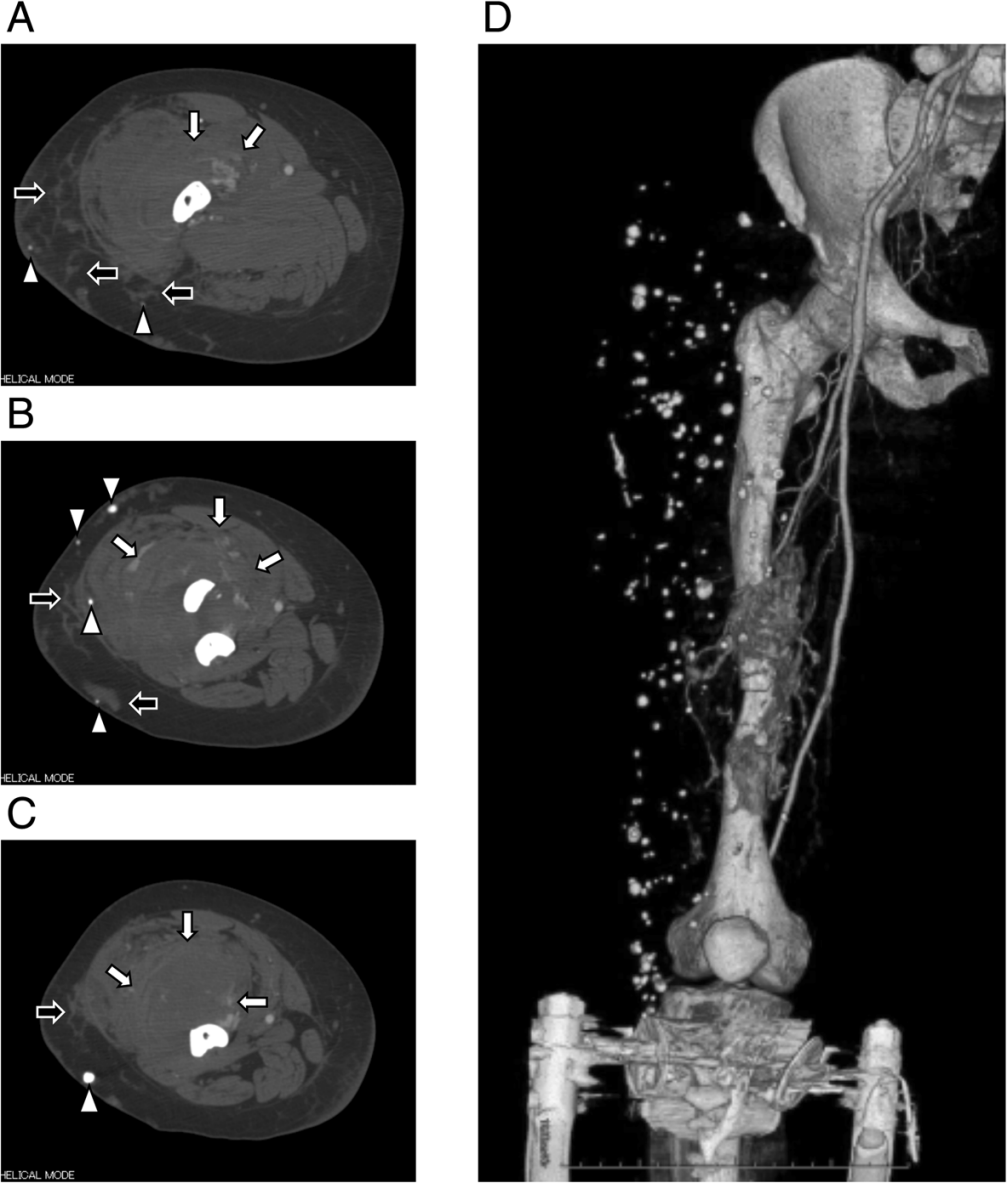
Preoperative enhanced computed tomography scans show large arteriovenous malformations (AVMs) in the quadriceps femoris (white arrows), which are partially present subcutaneously (black arrows). Numerous phleboliths (white arrowheads), massive bony erosion, and very narrow intramedullary canal are also observed (a: proximal site of fracture; b: fracture site; c: distal site of fracture). d Three-dimensional computed tomography angiography scan shows the AVMs are fed by the branches of the deep and superficial femoral artery
